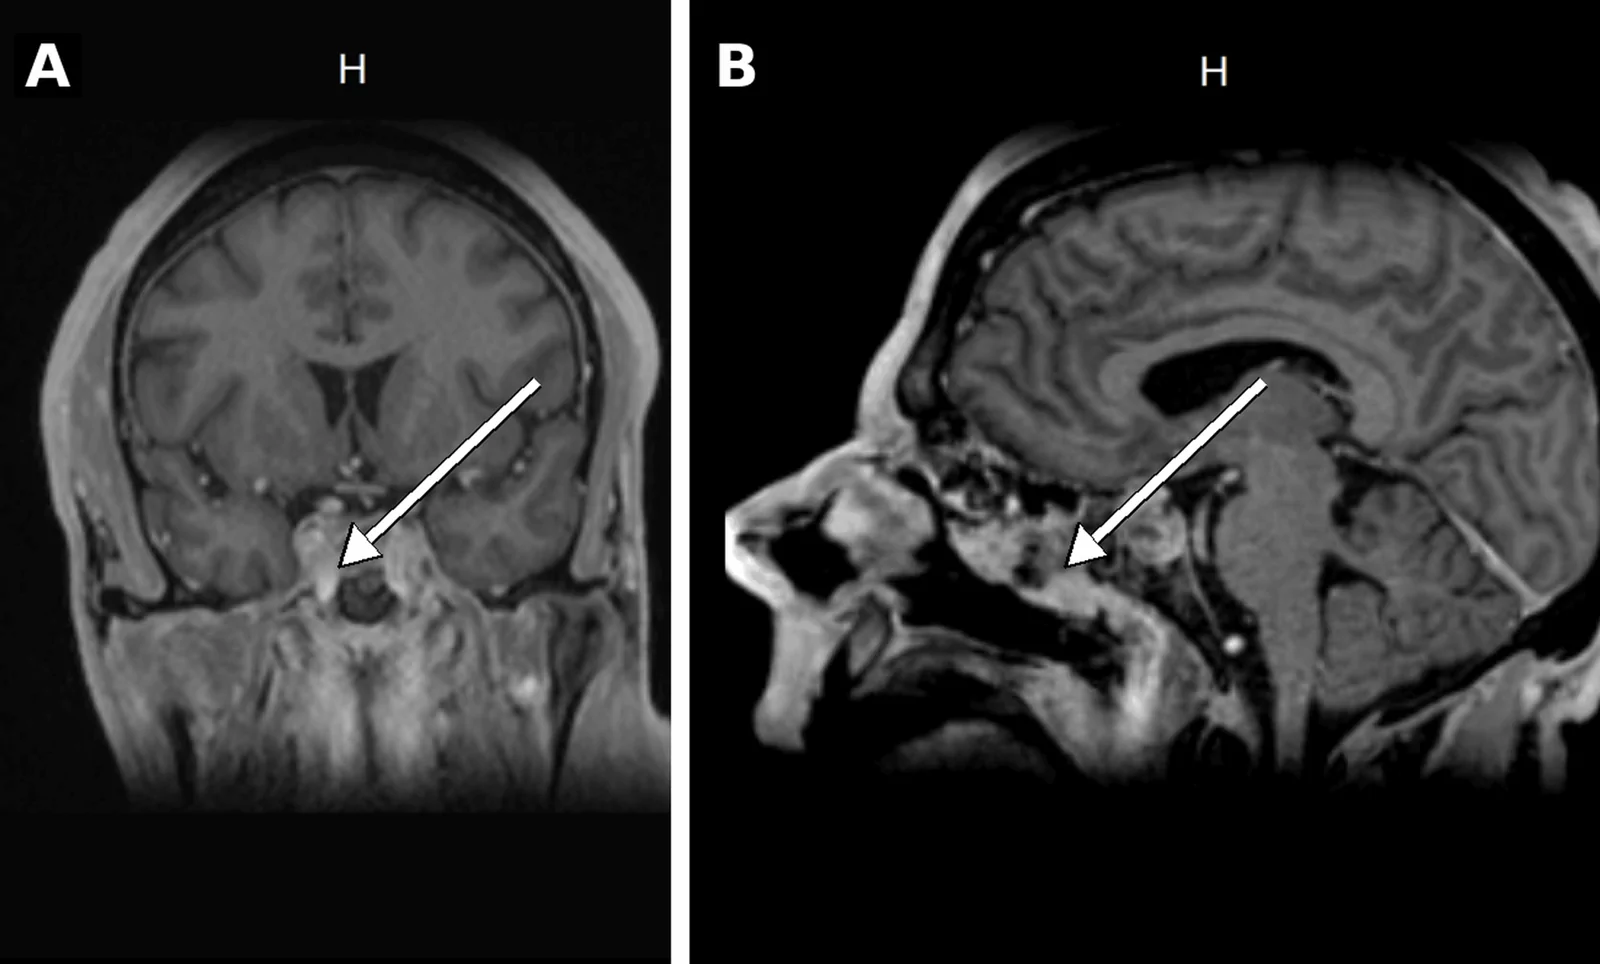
Immediate Postoperative Pituitary MRI on Postoperative Day 1 Showing Complete Tumor Resection Immediate postoperative pituitary MRI obtained on postoperative day 1, demonstrating expected postsurgical changes without evidence of residual tumor. (A) Coronal T1-weighted post-contrast image showing the surgical cavity (arrow) with postoperative material and no residual enhancing tumor. (B) Sagittal T1-weighted post-contrast image showing the postoperative sellar changes (arrow).
